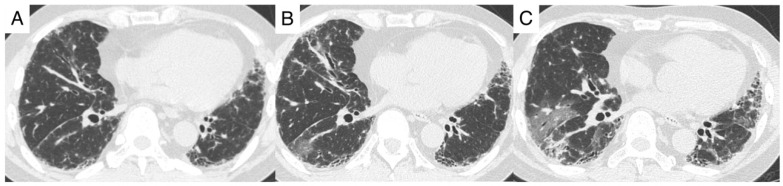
High-resolution computed tomography findings of Case 7. Dermatomyositis interstitial lung diseases, a 53-year-old man ((A): three months before onset of COVID-19, (B): onset of COVID-19, (C): one month after onset of COVID-19).
